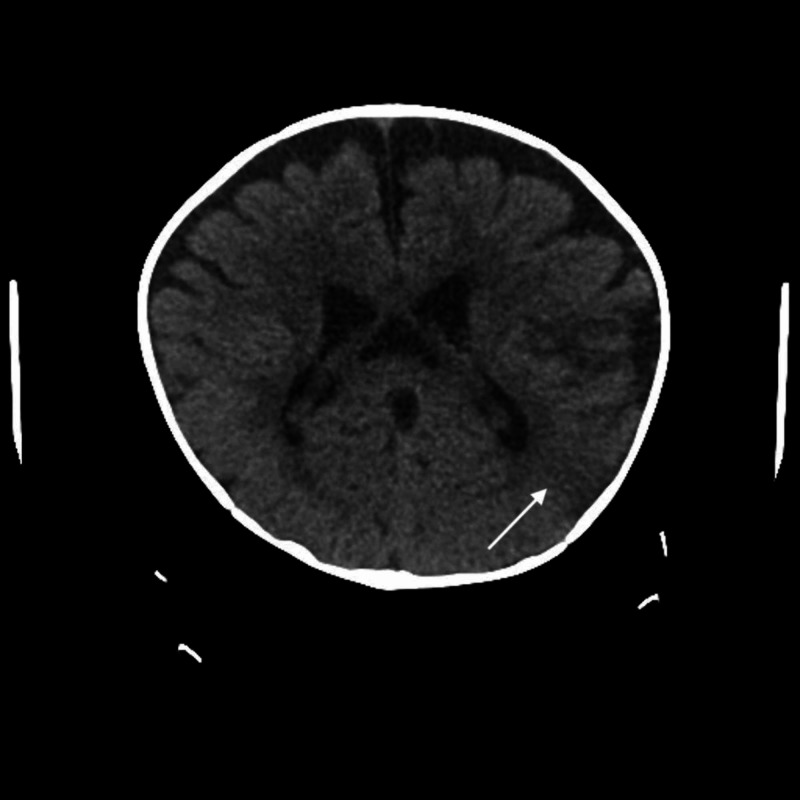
CT scan a 6-month, 3-week-old female demonstrating a left-posterior parietal lobe infarction.
